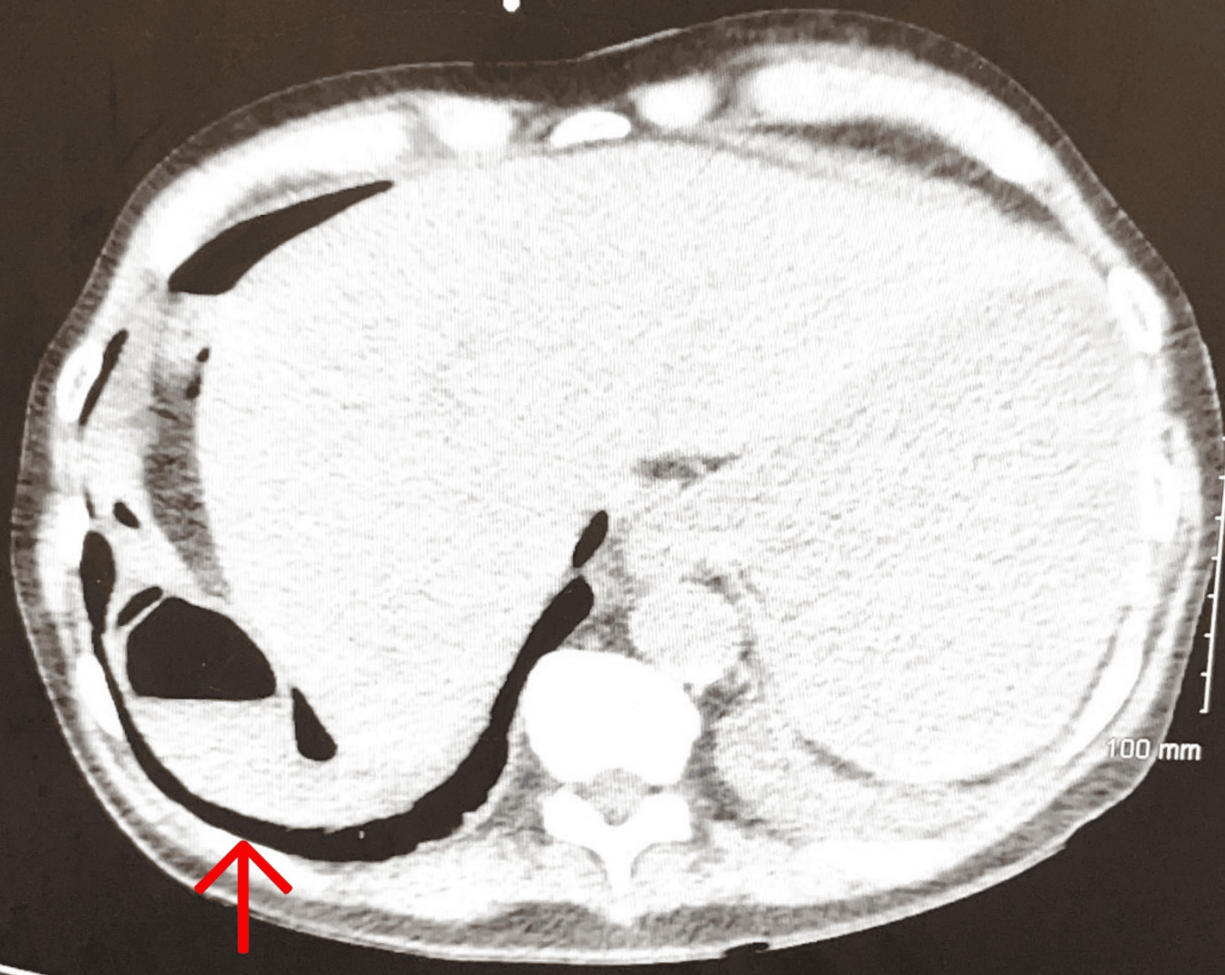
Transverse section of CT abdomen with IV contrast from emergency department showing small bowel obstruction with complex free fluid near the area of obstruction.
